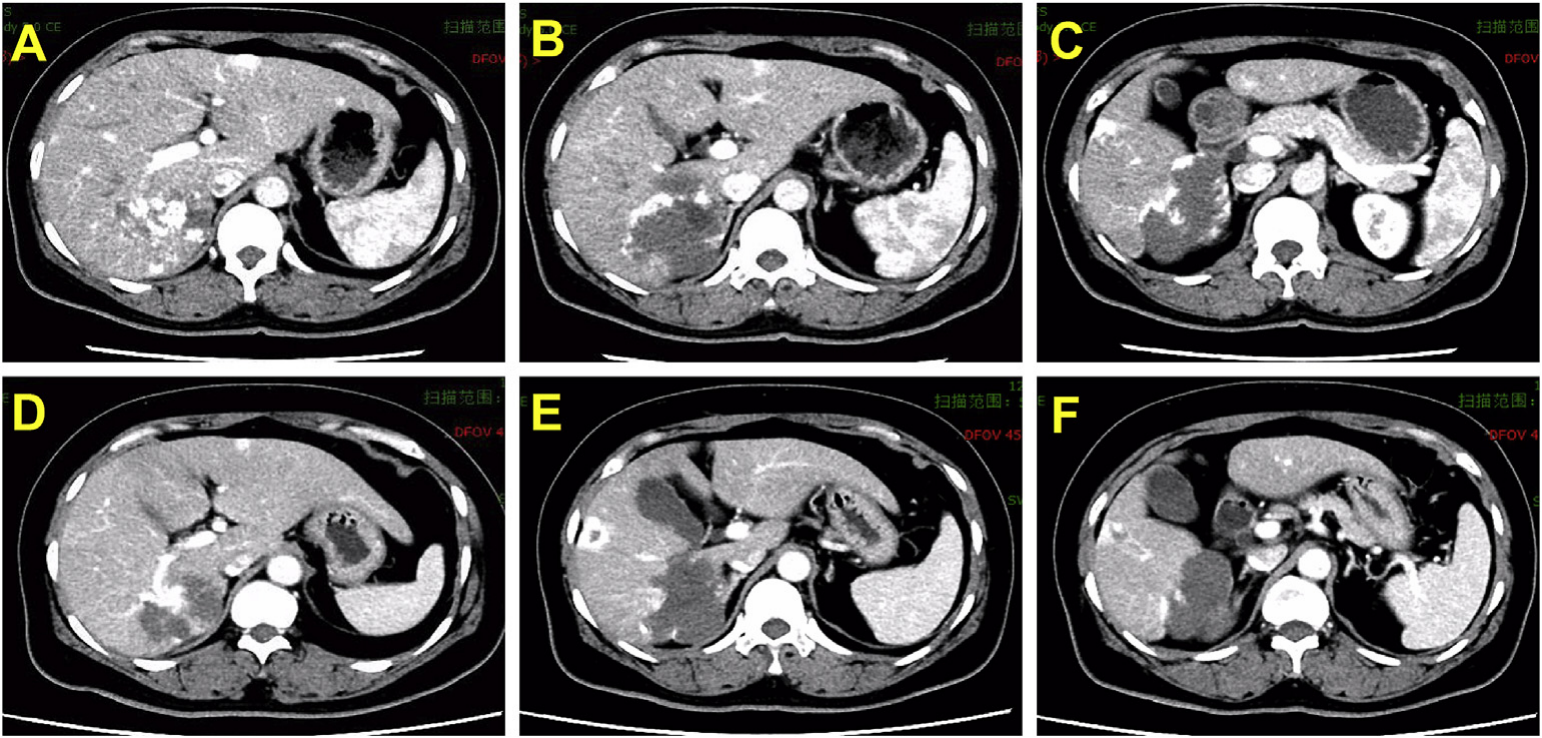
A 48-year-old woman had large hepatic hemangiomas in the right lobe (10.0 cm) as revealed by abdominal CT scans (A–C). Three months after ablation treatment, CT scans in the arterial phase showed that the hepatic hemangiomas in the right lobe had been completely ablated (D–F).
